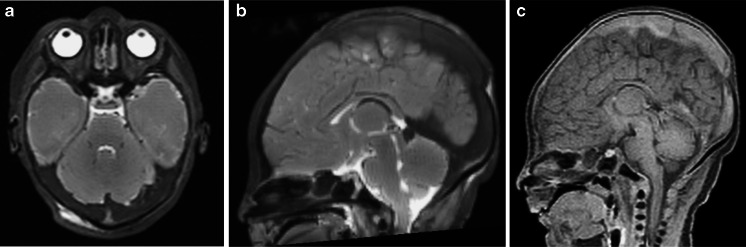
Venous sinus thrombosis. Axial (a) and sagittal T2-weighted (b) imaging, and sagittal T1-weighted (c) postmortem magnetic resonance imaging imaging of the brain in a neonatal death. These images show thrombosis of almost the entire venous sinuses, which is typically seen postmortem (rather than sedimentation as in Fig. 13). It is not currently possible to distinguish antemortem from postmortem venous sinus thrombosis. Note the absence of lens dislocation, which does not always occur (see Fig. 8)
